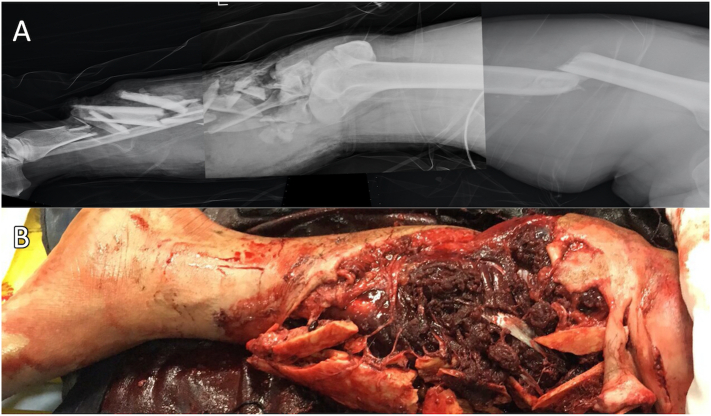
(A) The radiograph shows the femur shaft fracture and ipsilateral tibial plateau articular comminuted fracture extended to the diaphysis. (B) A large soft tissue defect around the knee is evident.
